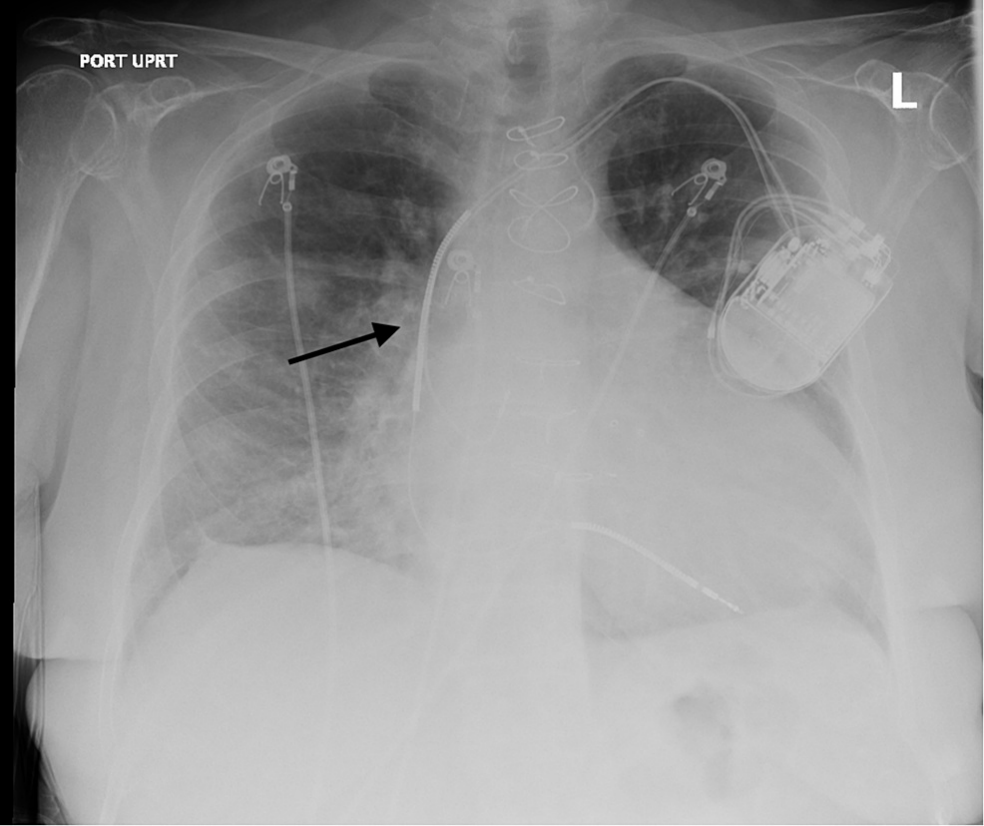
Chest X-ray: arrow showing interstitial infiltrates in the right lung
